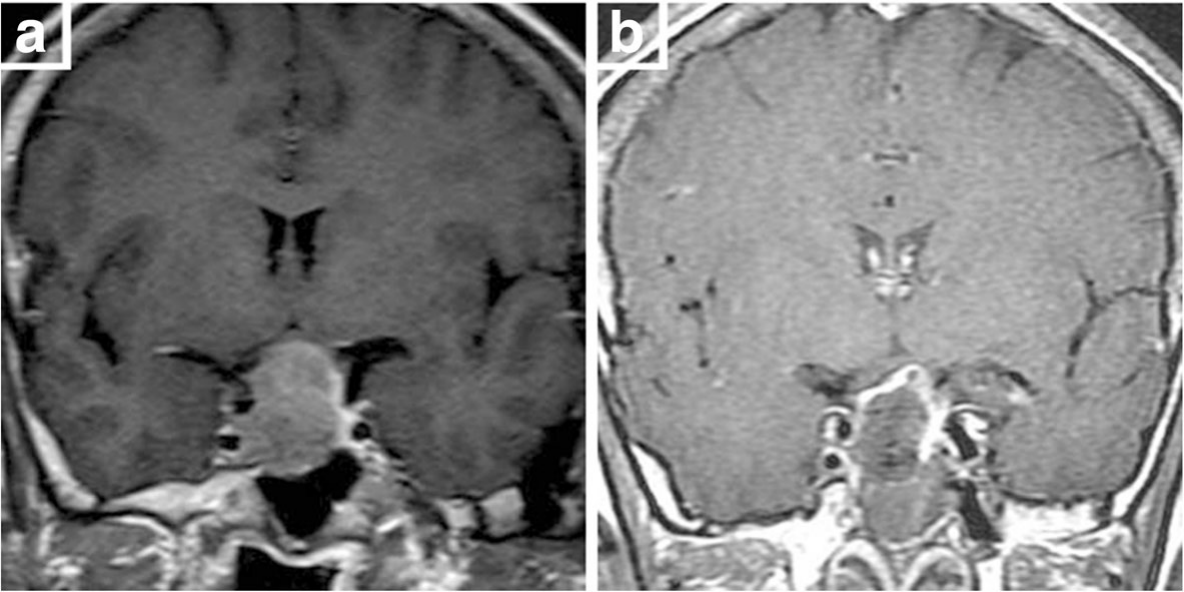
a Preoperative MRI shows a GH pituitary macroadenoma of Knosp grade III. b Postoperative MRI shows total tumor resection. Endocrinal evaluation on the third postoperative month shows: GH: 0.57 ng/ml, IGF-1: 207 ng/ml (normal range, 94 ~ 284 ng/ml)
